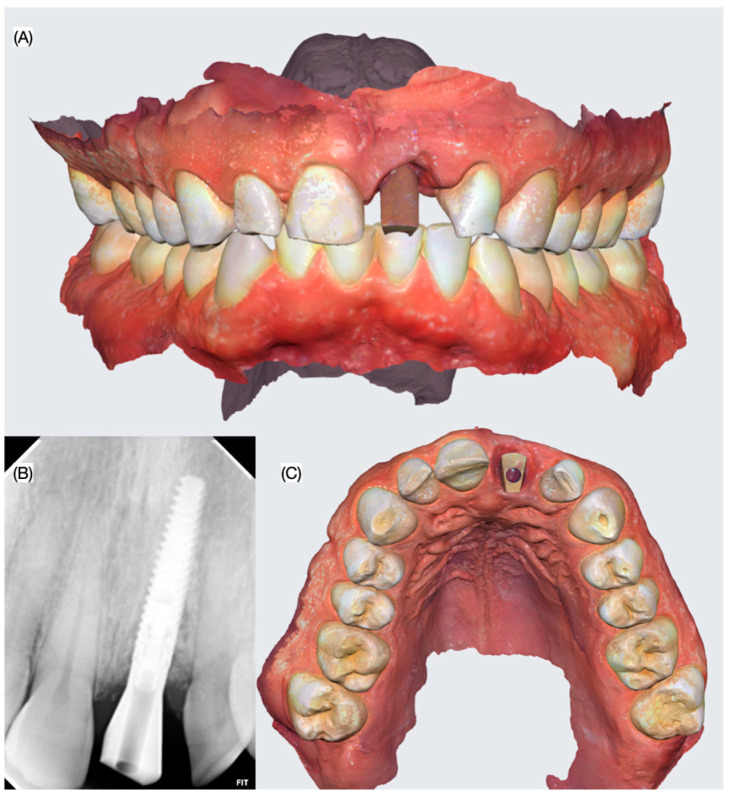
Final digital impression. (A) Frontal view, (B) periapical radiograph and (C) incisal view of the scan body used for digital impression.
